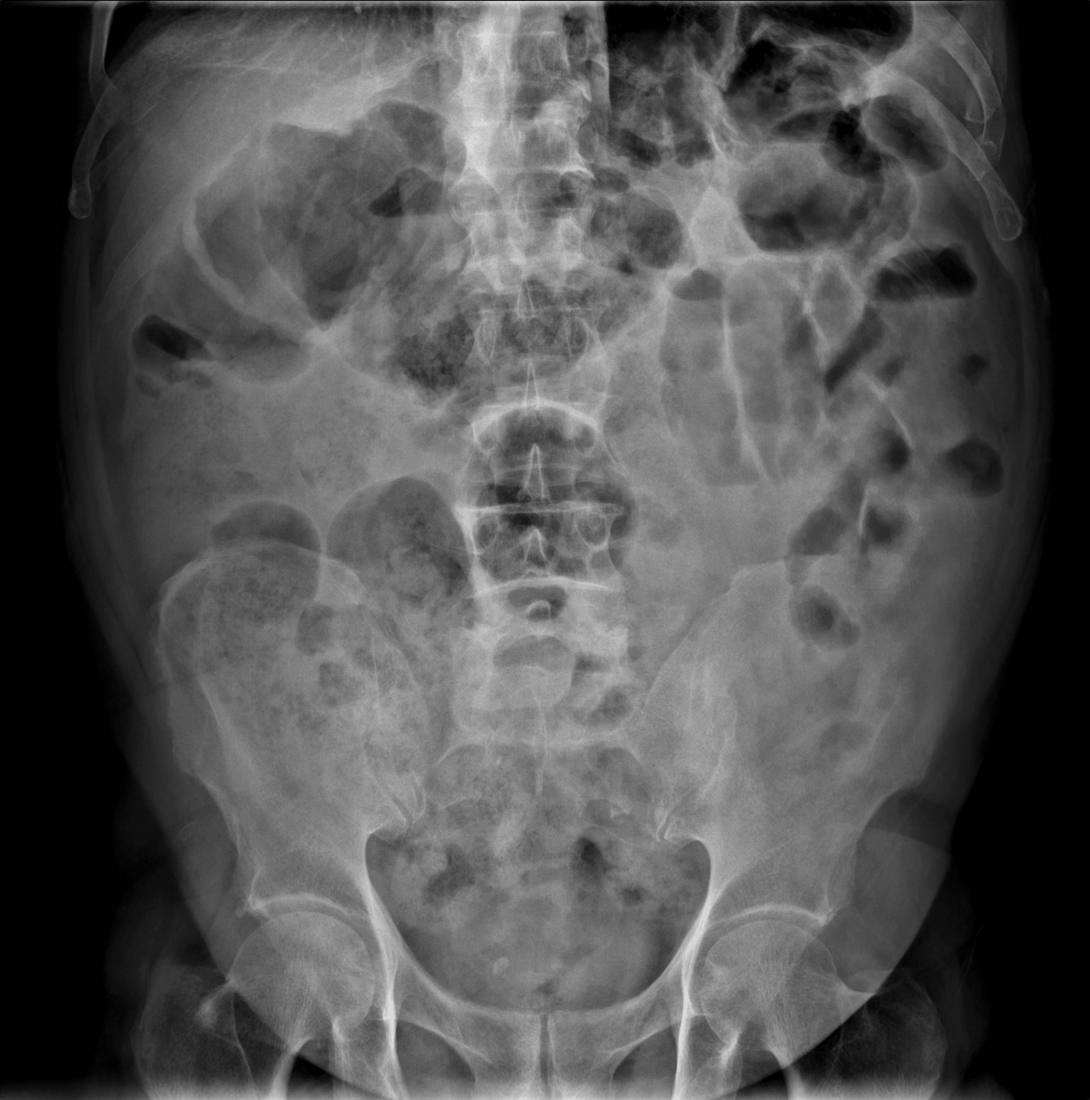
Plain abdominal radiography compatible with functional intestinal obstruction
